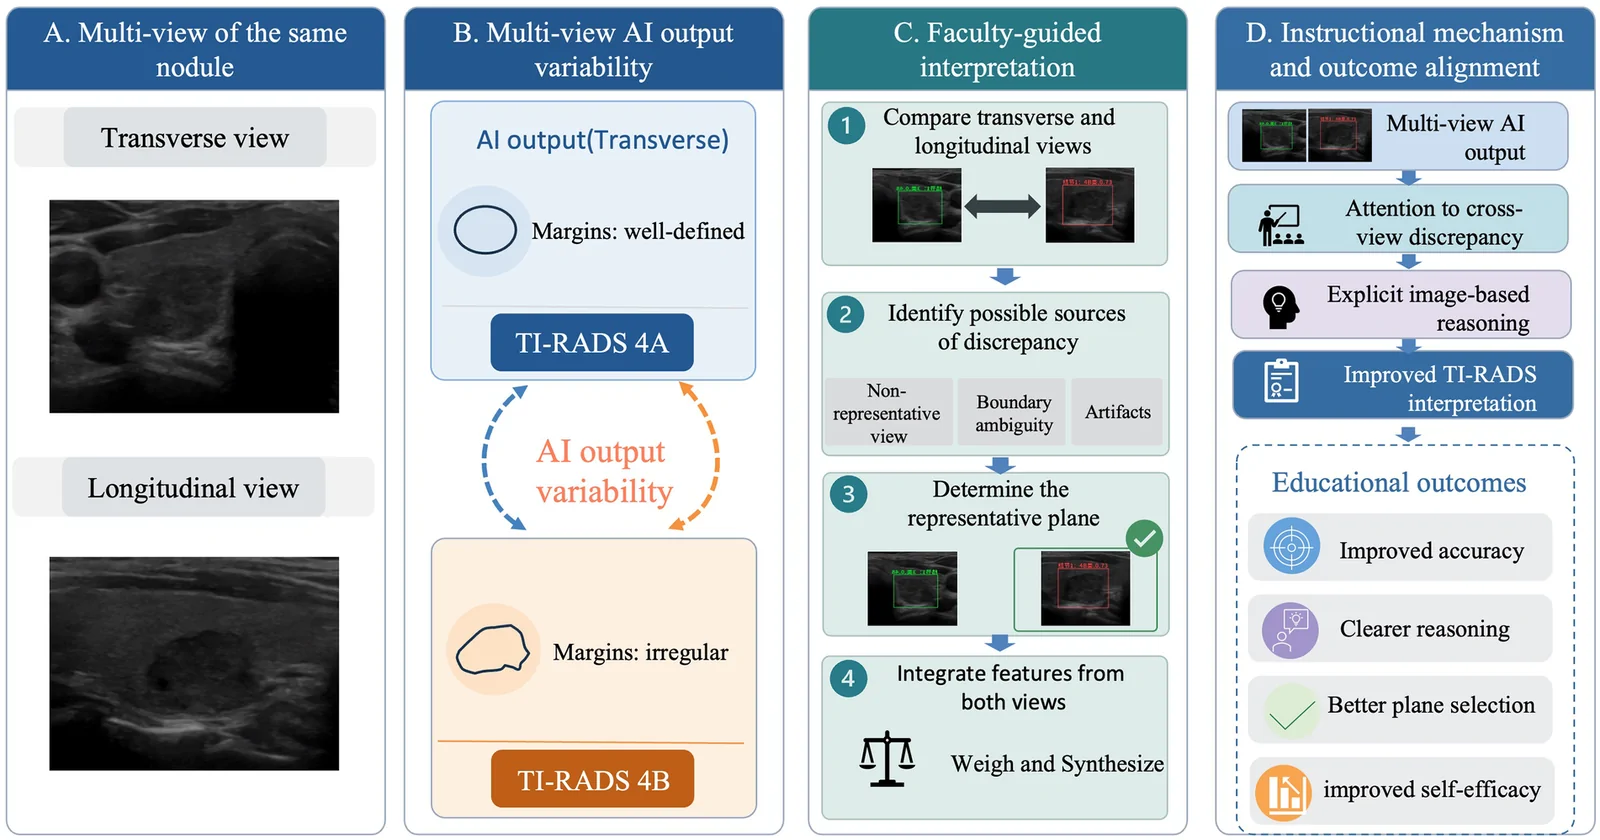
Proposed instructional pathway of using multi-view AI output variability in TI-RADS training. (A) Transverse and longitudinal ultrasound images show two orthogonal views of the same thyroid nodule. (B) Multi-view AI output variability. (C) Faculty guided residents through four sequential steps. (D) The proposed instructional pathway links exposure to multi-view AI output variability with attention to cross-view discrepancies, explicit image-based reasoning, and improved TI-RADS interpretation, with accuracy, reasoning clarity, representative-plane selection, and self-efficacy as educational outcomes. AI outputs served as discrepancy signals and did not constitute the reference standard or answer key. AI, artificial intelligence; TI-RADS, Thyroid Imaging Reporting and Data System.
